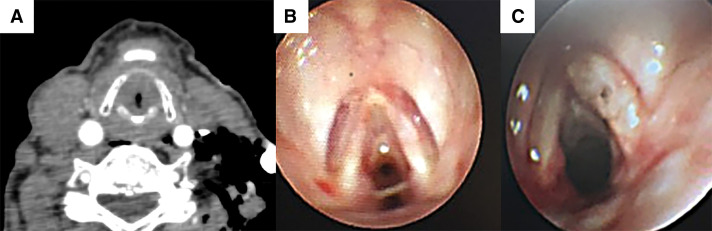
(A, B) Cervical CT and bronchoscopy images obtained before coring out show a circumferential membranous structure below the glottis that caused 90% stenosis. (C) Bronchoscopy images obtained the day after coring out show no remnants of the pseudomembrane, and the stenosis has been resolved.
